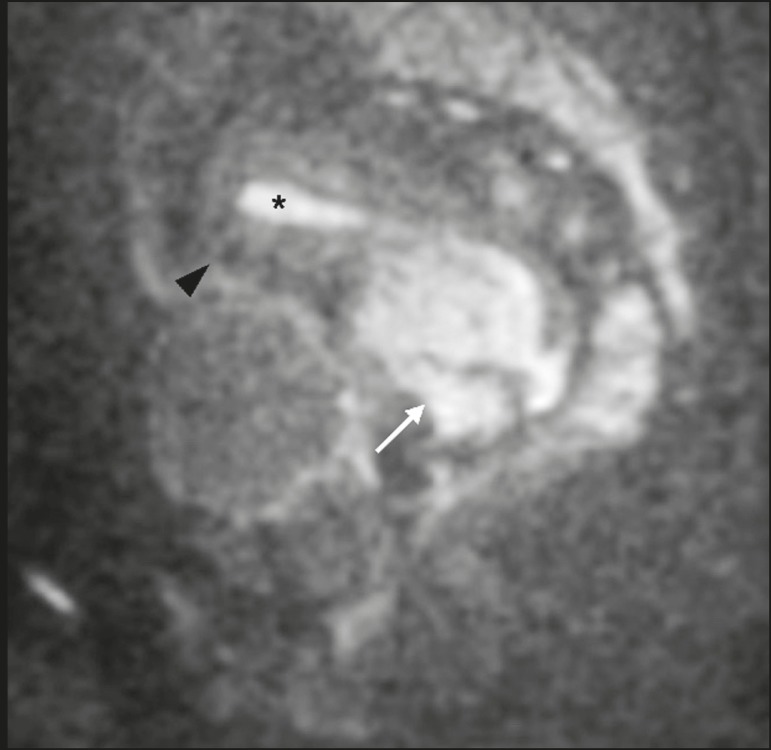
MRI of a female patient with cervical cancer. Sagittal DWI at b = 1000
